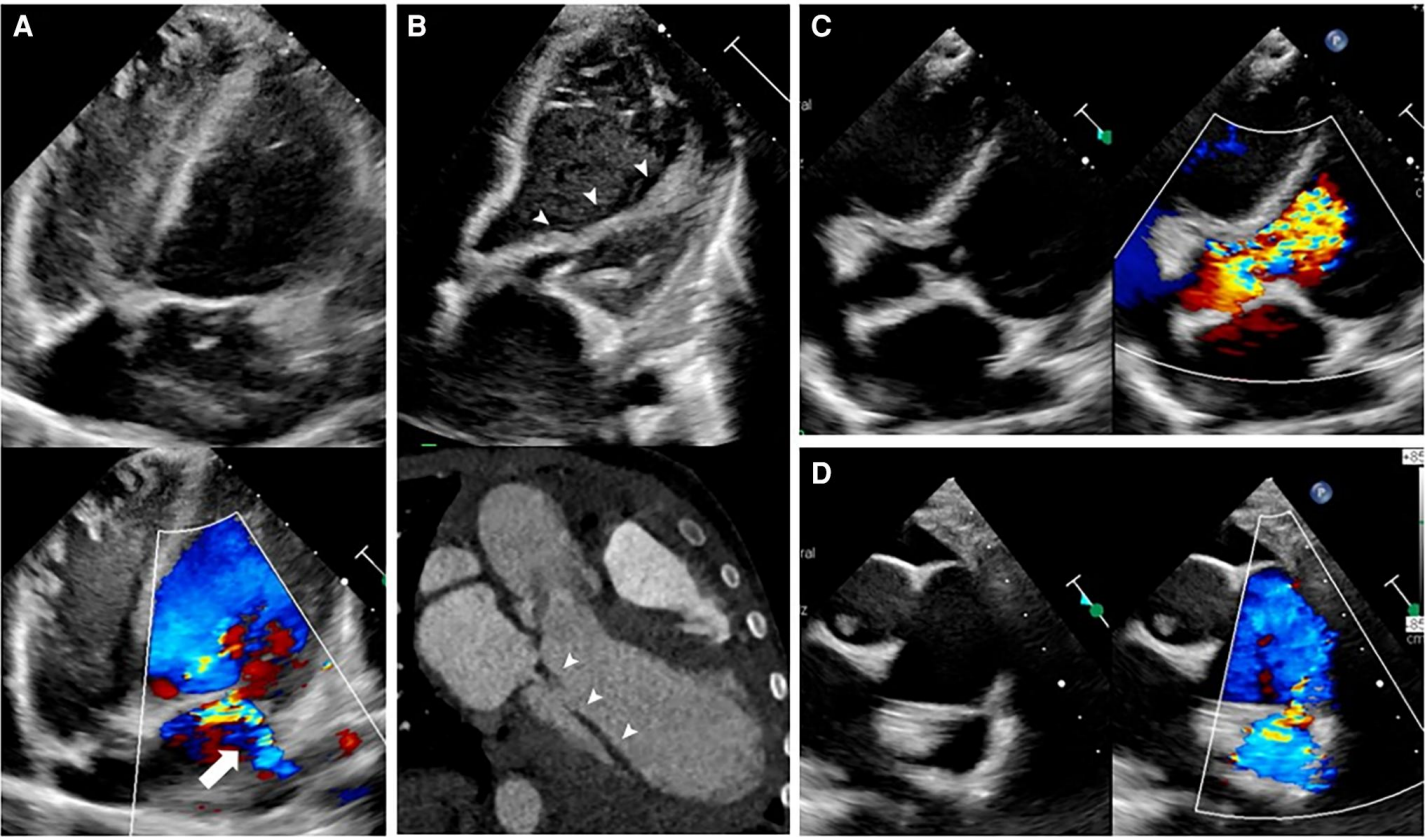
(*A*) Dual four-chamber view showing mitral regurgitation (*white arrow*). (*B*) Echocardiography view (*above*) and tomographic view (*bottom*) demonstrating a hammock mitral valve (*white arrows heads*). (*C*) Dual five-chamber view revealing severe acute regurgitation (*blue arrow*). (*D*) Dual view demonstrating PDA (*yellow arrow*).
